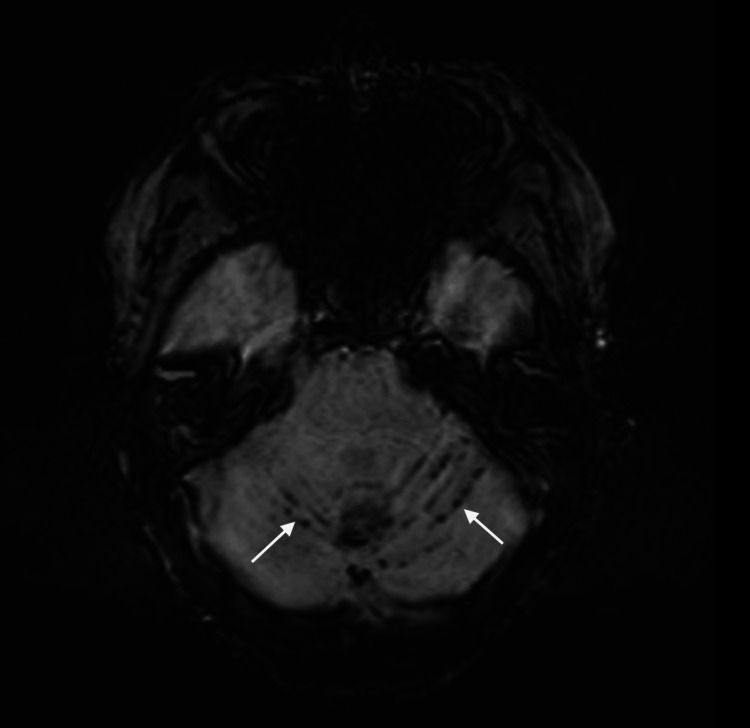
Case 1: MRI of the brain, susceptibility-weighted image (SWI) SWI confirms hemosiderin deposition involving the cerebellar vermis and bilateral cerebellar hemispheres (arrowed).
